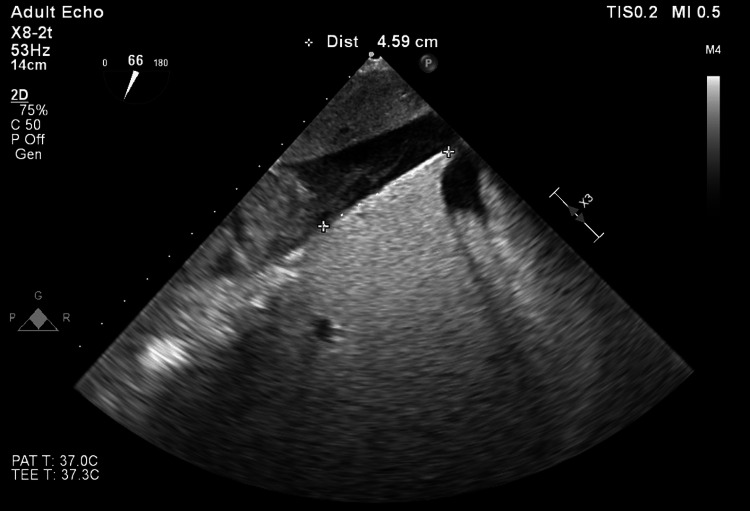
Intraoperative TEE demonstrating IVC thrombus with liver in long axis. TEE: Transesophageal echocardiography; IVC: Inferior vena cava
